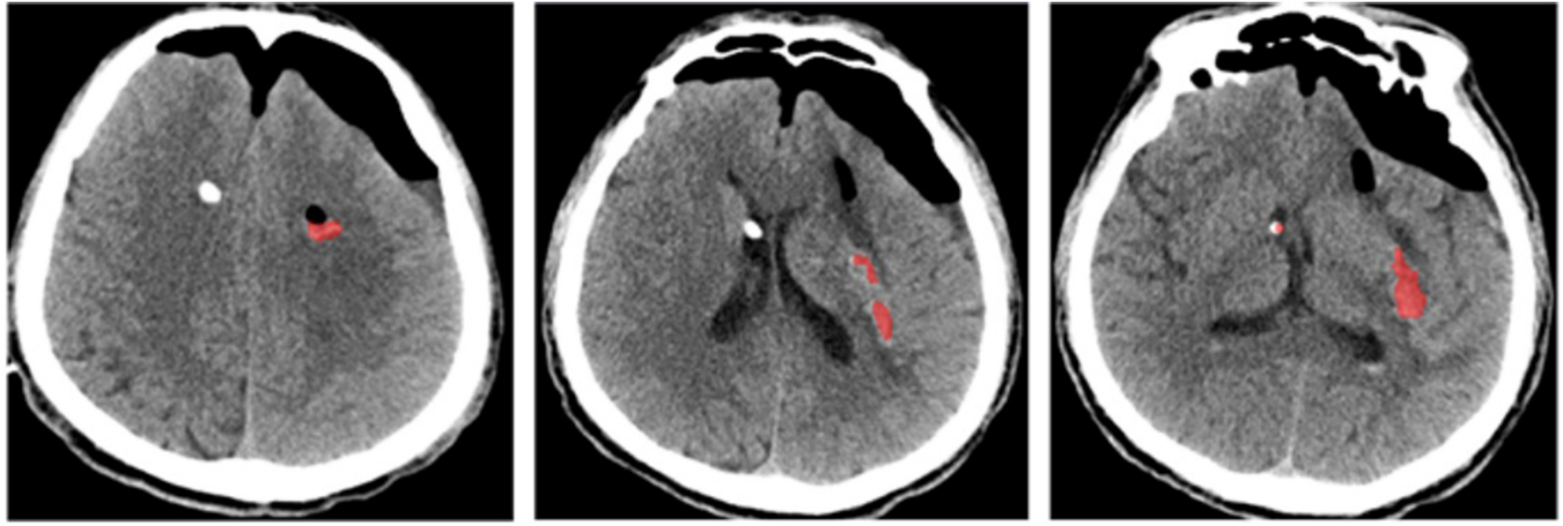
Postoperative review. Head CT examination 12 h after operation showed residual blood volume of 5.3 ml and hematoma clearance rate of 95.28%.
